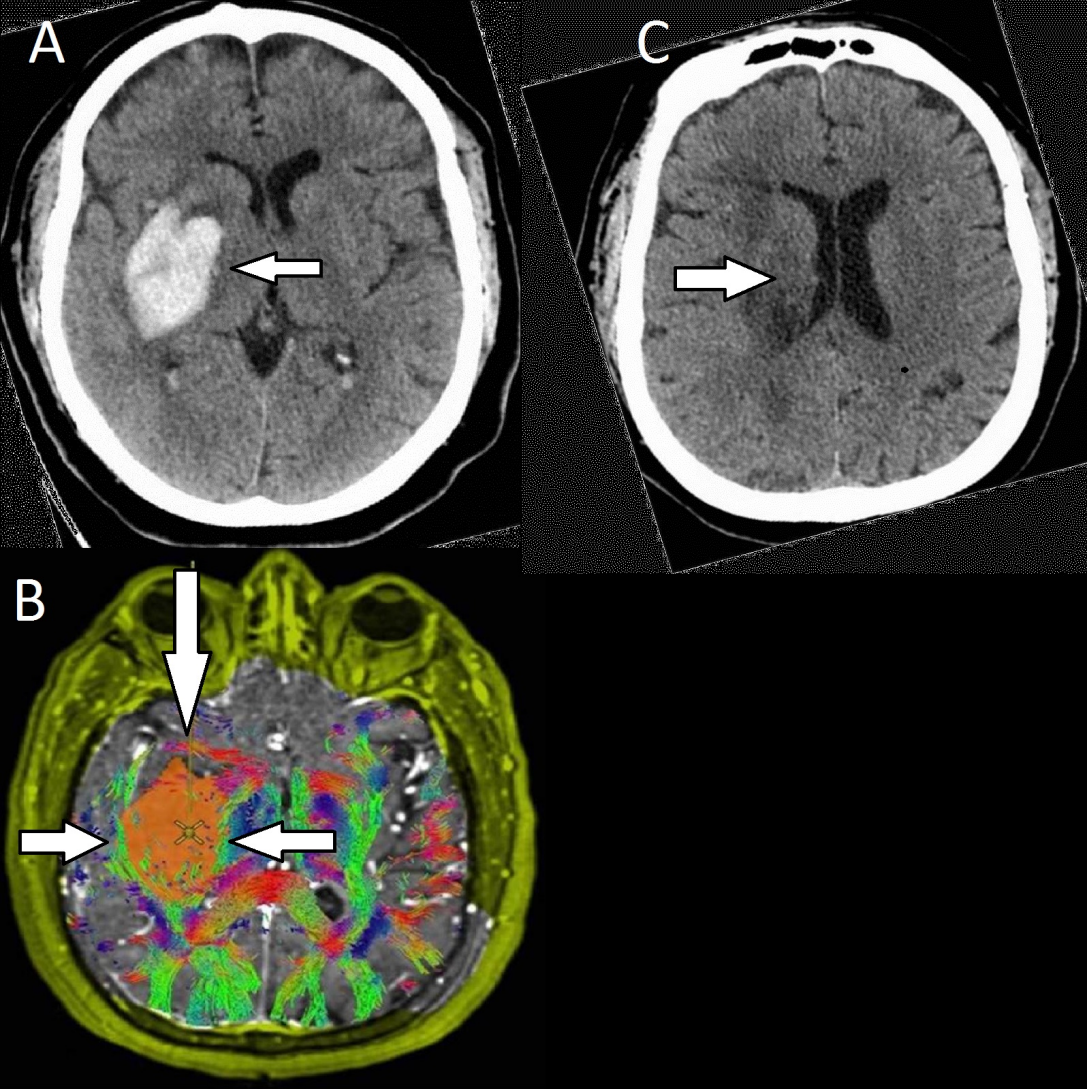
Preoperative and postoperative images for patient three with a basal ganglia hemorrhage A: CT scan of the head showed a 4.8 x 3.0 x 4.0 cm right intraparenchymal hemorrhage (arrow) with surrounding edema. B: DTI revealed medial displacement of the internal capsule (leftward arrow) and lateral displacement of the SLF (rightward arrow). An anterior corridor was used to approach the hematoma (downward arrow). C: A postoperative CT scan of the head showing evacuation of the hematoma (arrow).
